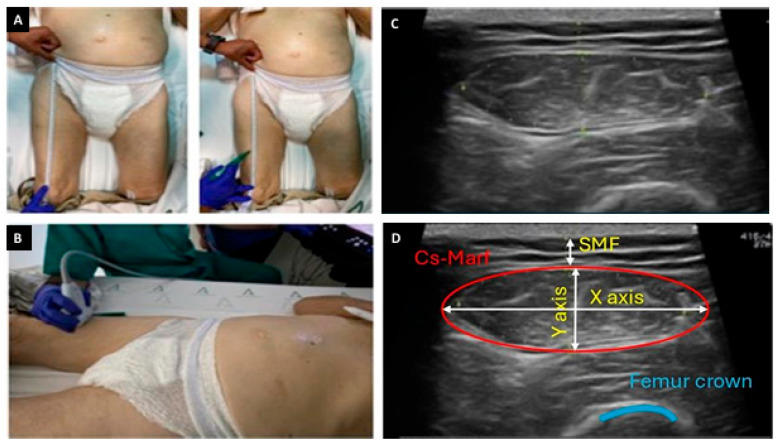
Systematic nutritional ultrasound study of the anterior quadriceps rectus femoris (QRF) muscle. The patient was in the supine decubitus position with the headrest at 0° with both legs extended and relaxed. An imaginary line was drawn between the anterosuperior iliac crest and the upper edge of the patella (A), and the transducer was placed in a transverse position to the axis of the leg in the lower third to measure the cross-sectional area of the anterior QRF (B). The QRF muscle and its ultrasonographic measurements (C,D). CS-MARF: cross-sectional muscle area of the rectus femoris; SMF: supramuscular fat.
